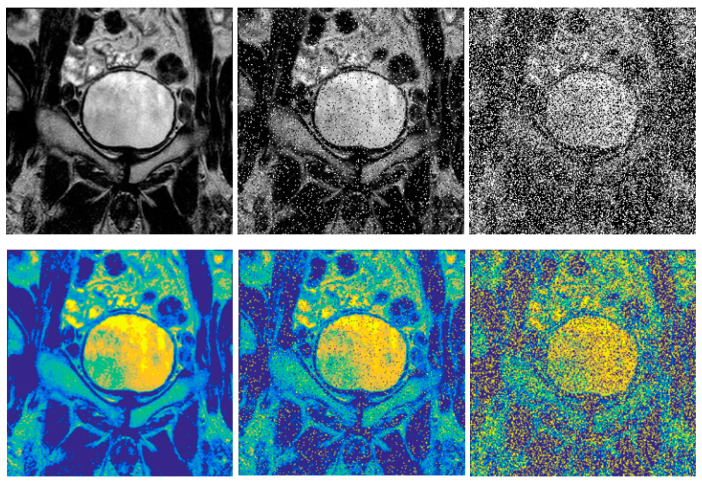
Extract of MR images: native MR image (up left), Salt and Pepper: d = 0.1 (up middle), and d = 0.5 (up right). Regional segmentation based on Fuzzy thresholding with 8 classes: segmentation of native image (down left), Salt and Pepper: d = 0.1 (down middle), and d = 0.5 (down right).
